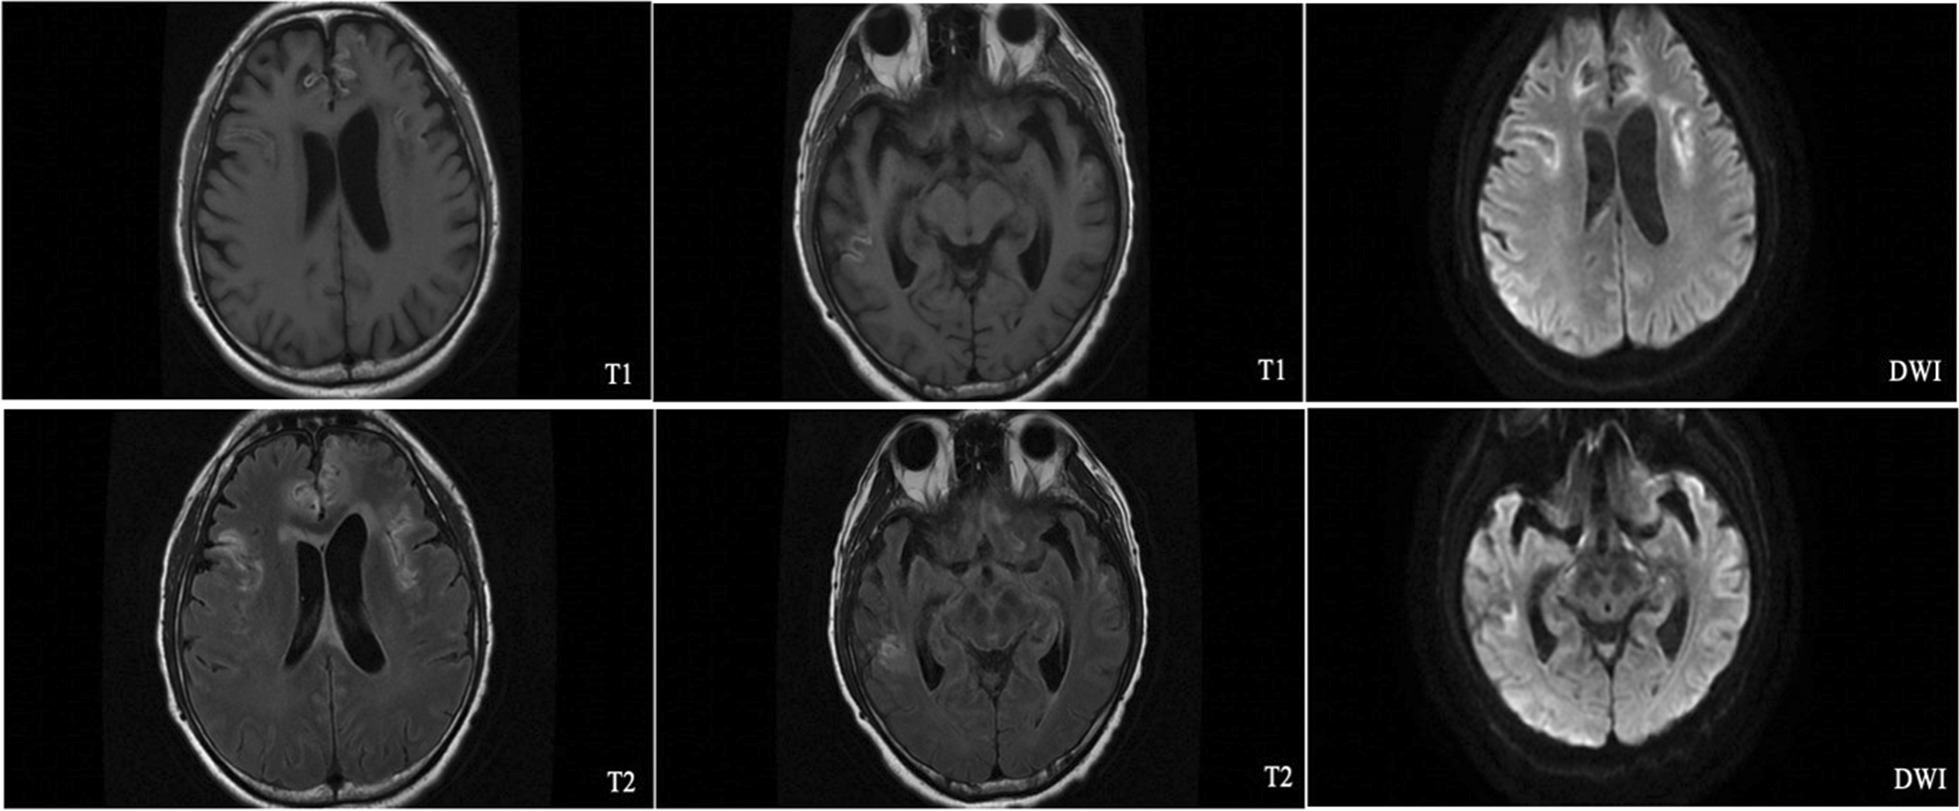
Brain MRI scan revealed multiple lesions and lamellar necrosis in the parietal lobe of the frontotemporal island and the cortex of the left cerebellar hemisphere, also we observed hydrocephalus in several lesions, accompanied by interstitial edema
